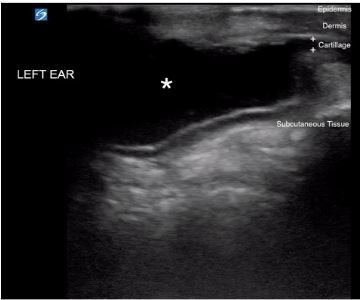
Ultrasound image (transverse view) of the left ear demonstrating an anechoic fluid collection (asterisk) within the cartilaginous layer (plus sign) of the pinna.
